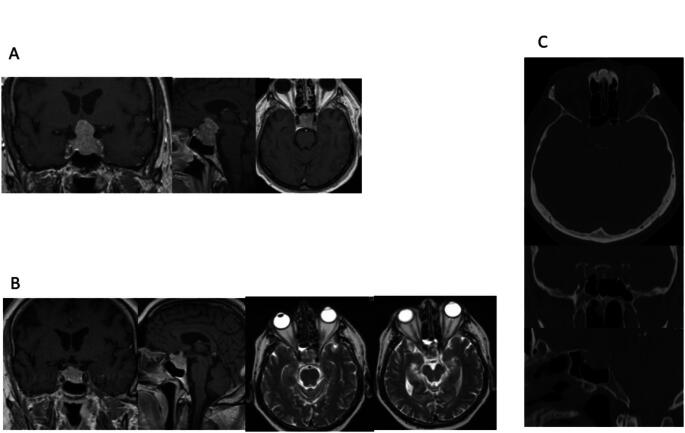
Imaging studies of patient 3 (66-year-old man). A T1-weighted contrast enhanced MRI studies demonstrate a macroadenoma with para- and suprasellar expansion through the sellar diaphragm. B T1-weighted contrast enhanced MRI studies and T2-weighted MRI studies 6 weeks after cabergoline therapy show considerable shrinkage of the tumor. Note the fluid level in the posterior ethmoid cells on the right side. C CT imaging in bone window show the bony destruction of the upper clivus with tumor invasion into the ethmoid sinus and the nasal cavity
